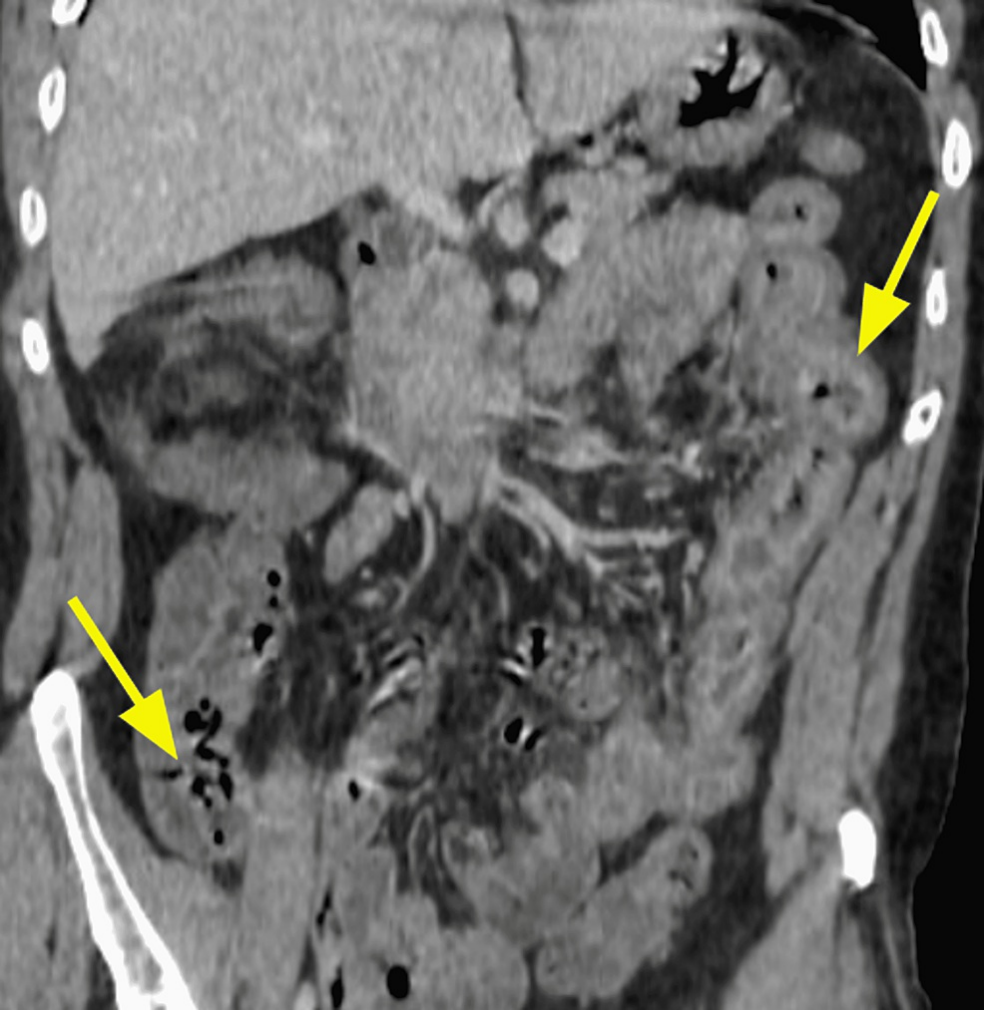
Contrast-enhanced CT image for Case 1 (coronal plane) demonstrating thickening of the caecum, the right and left large bowel wall, and increased mucosal enhancement (arrows)
